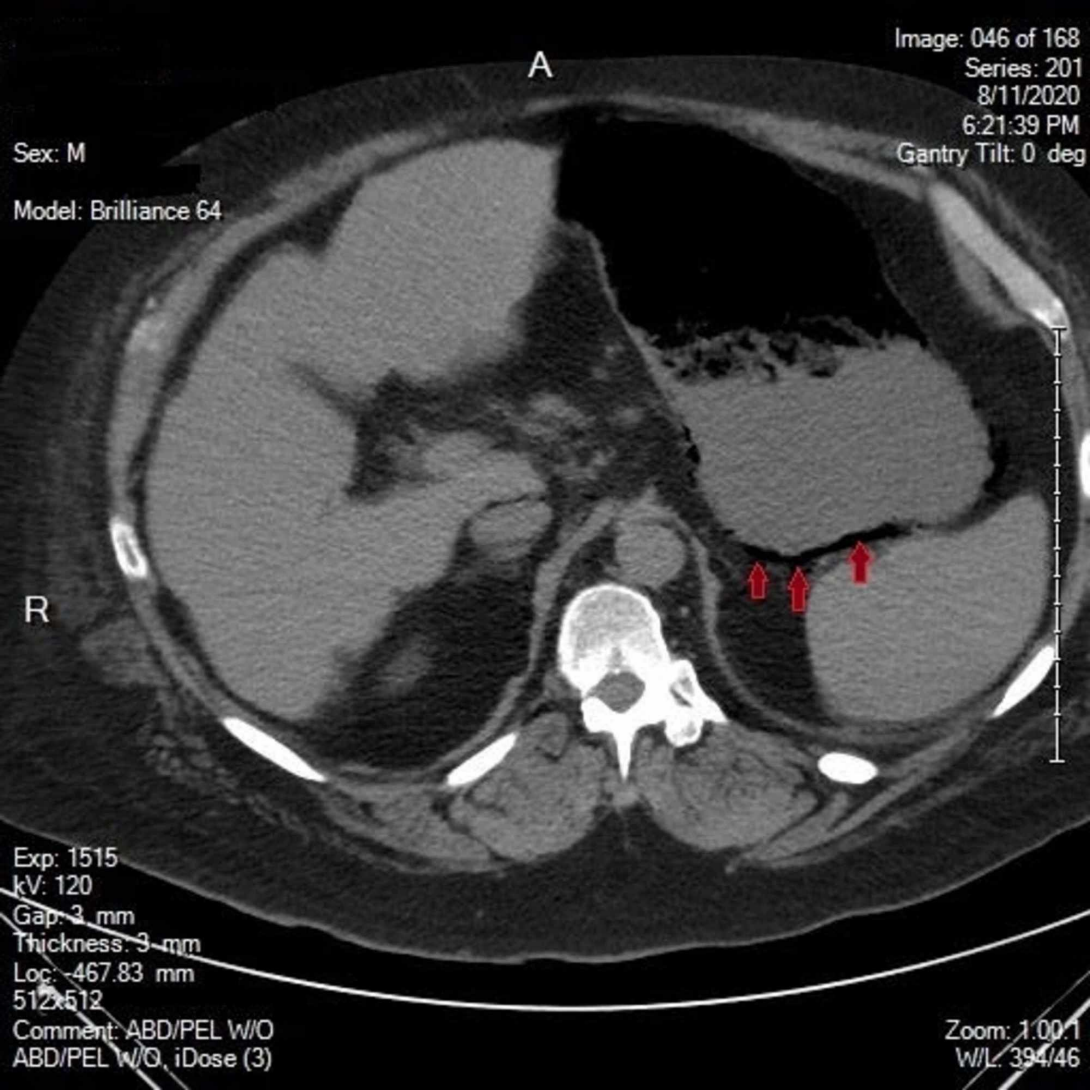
CT abdomen/pelvis demonstrating air within the wall of the stomach
